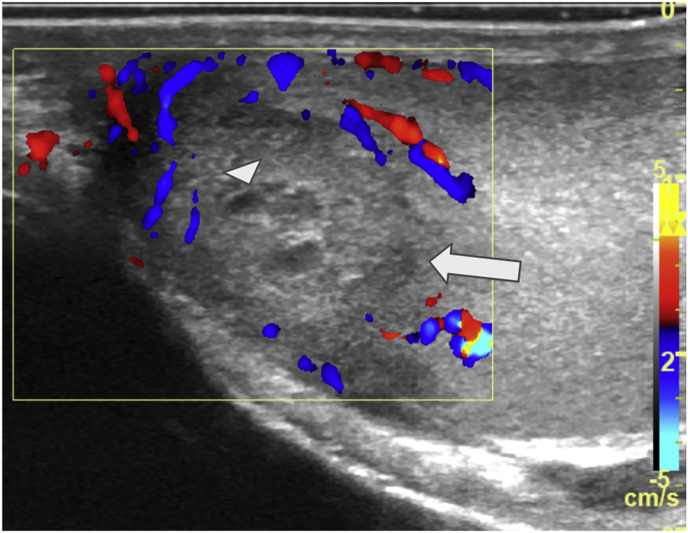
Testicular ultrasound - Isoechoic nodule with a hypoechoic halo (arrow) and a heterogeneous center (arrowhead). Doppler ultrasonography demonstrated peripheral vascularization.
